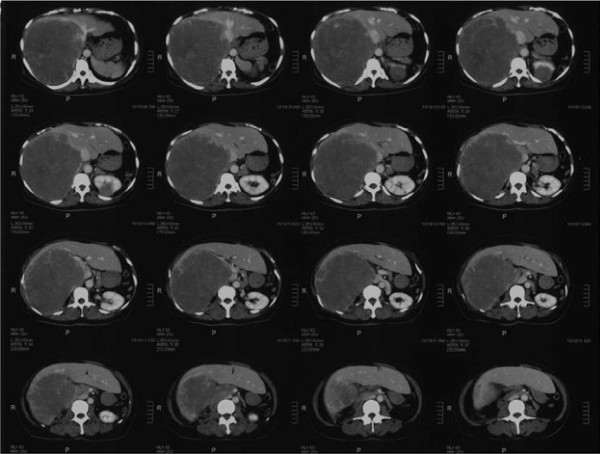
Abdominal computed tomography before sunitinib administration. A massive liver metastasis is present in the right hepatic lobe, and there is also a left renal metastasis.
